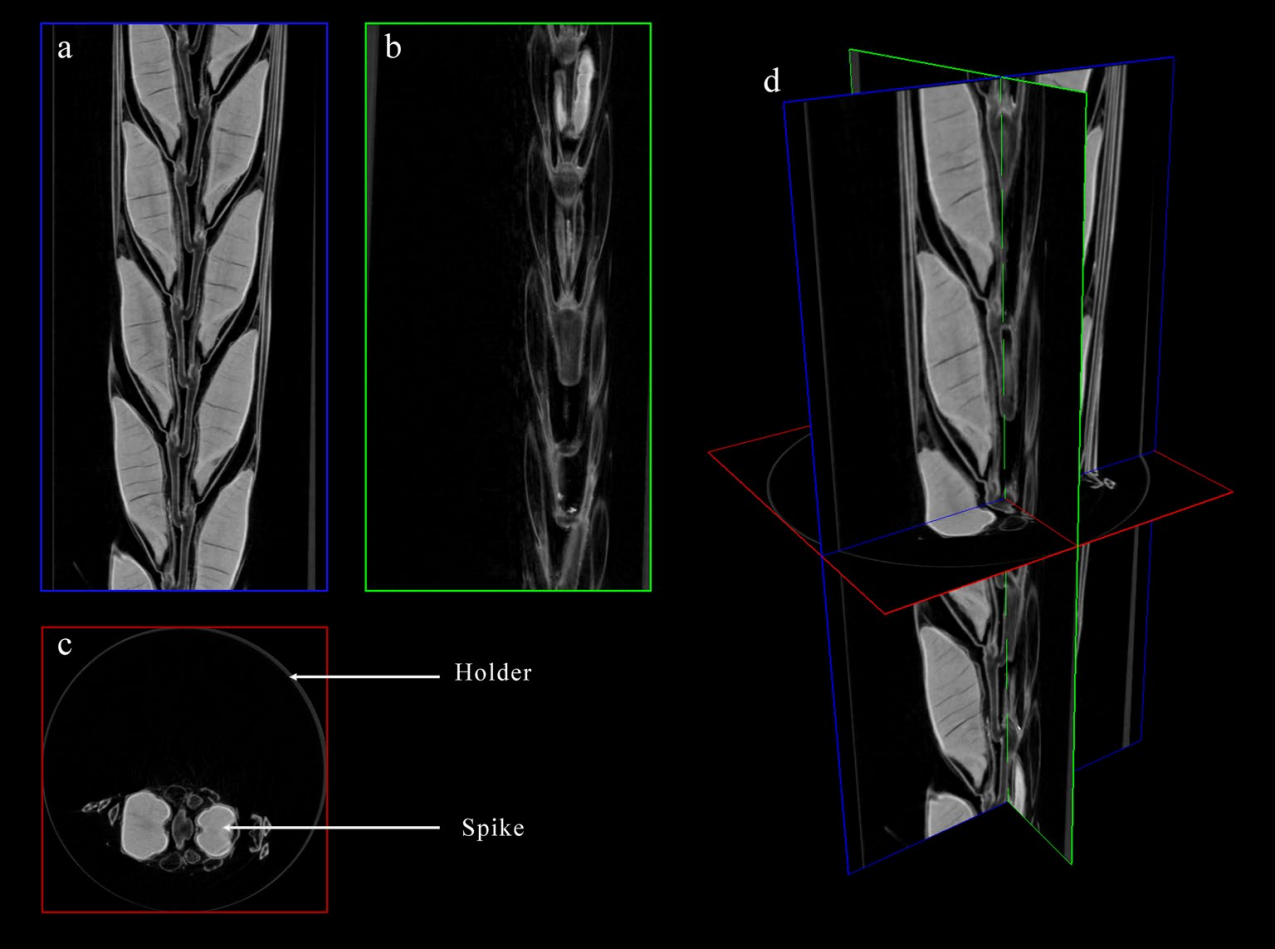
CT 3D reconstructed high resolution slice images of a barley spike (22 µm/pixel). a Coronal section. b Sagittal section. c Transverse section. d Three section stacked image
